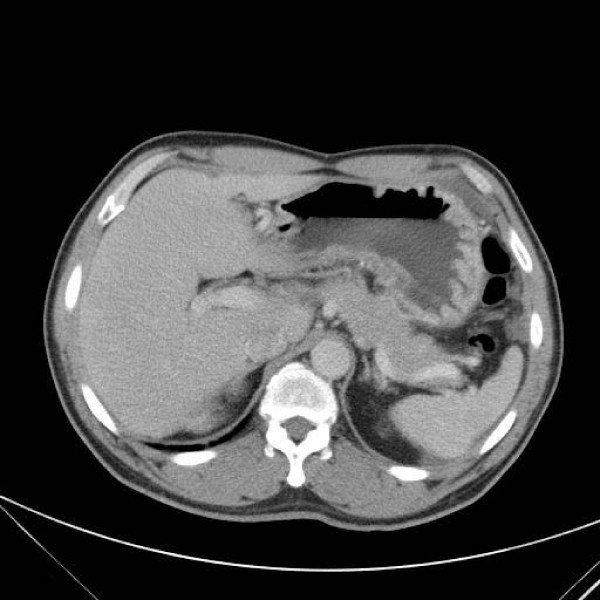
CT scan of abdomen – postoperative 17 months, compared with Fig 2. No local recurrence or metastatic lesion is identified.
